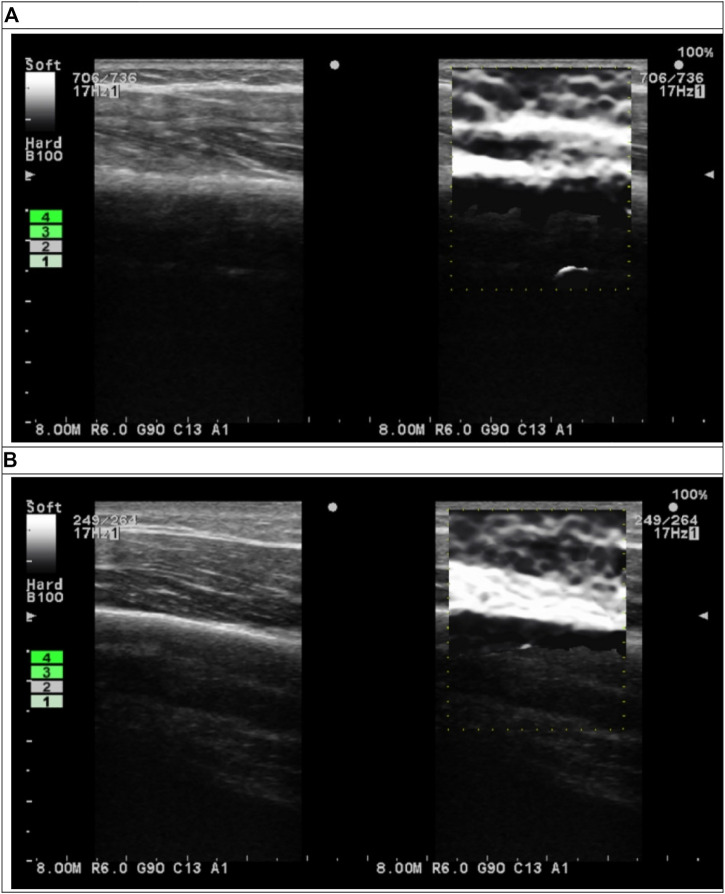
B-mode (left subfigures) and elastographic (right subfigures) ultrasound images of triceps (A) before and (B) after cupping therapy.
